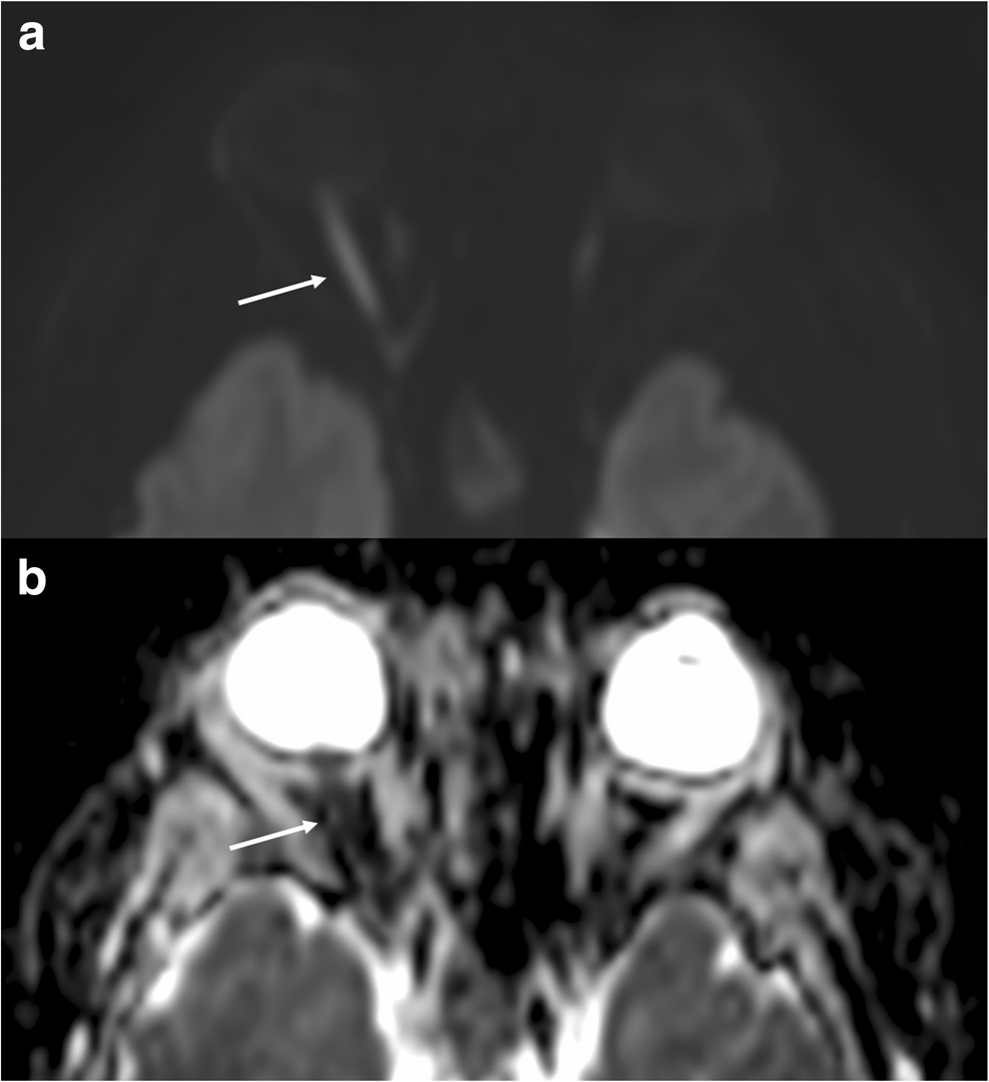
Optic neuritis. High DWI signal (a) and low ADC value (b) representing restricted diffusion in the right optic nerve in a patient with non-specific optic neuritis
